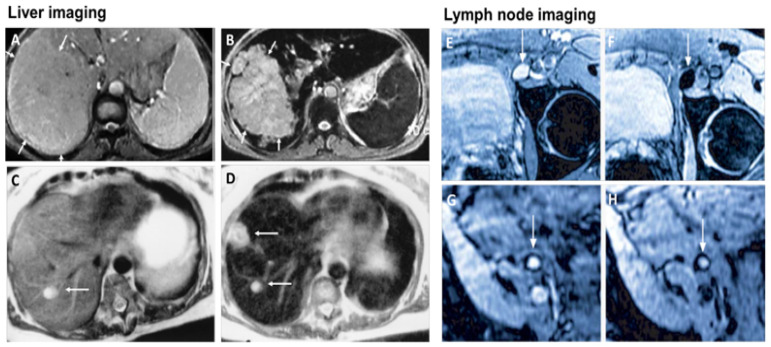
Superparamagnetic iron oxide NPs being used in liver imaging and lymph node imaging. (A,B): T2-weighted MR image of a liver with a large hepatocellular carcinoma before (A) and after (B) the administration of SPION. The lesion is demarcated with arrows. (C,D): Standard (C) and SPION-based contrast-enhanced (D) MR imaging of liver metastasis in a patient with colorectal cancer. After administration of ferumoxide SPION, a second metastasis becomes visible on T2-weighted MR image. (E,H): Lymph node in left iliac region (arrow), with and without metastatic infiltration. T2-weighted images before (E,G) and 24 h after (F,H) administration of ferumoxtran. Lymph node (arrow) appears bright before injection of UPIO (E,G). One day after injection, a signal loss in the lymph node (arrow) due to high UPIO macrophage uptake can be observed, thus indicating functionality and no metastasis (F). Conversely, in the lower panel, the lymph node (arrow) stays bright, indicating no trafficking of USPIO and thus metastatic colonization (H). Reprinted with permission from Ref. [30]. Copyright 2020 Elsevier.
